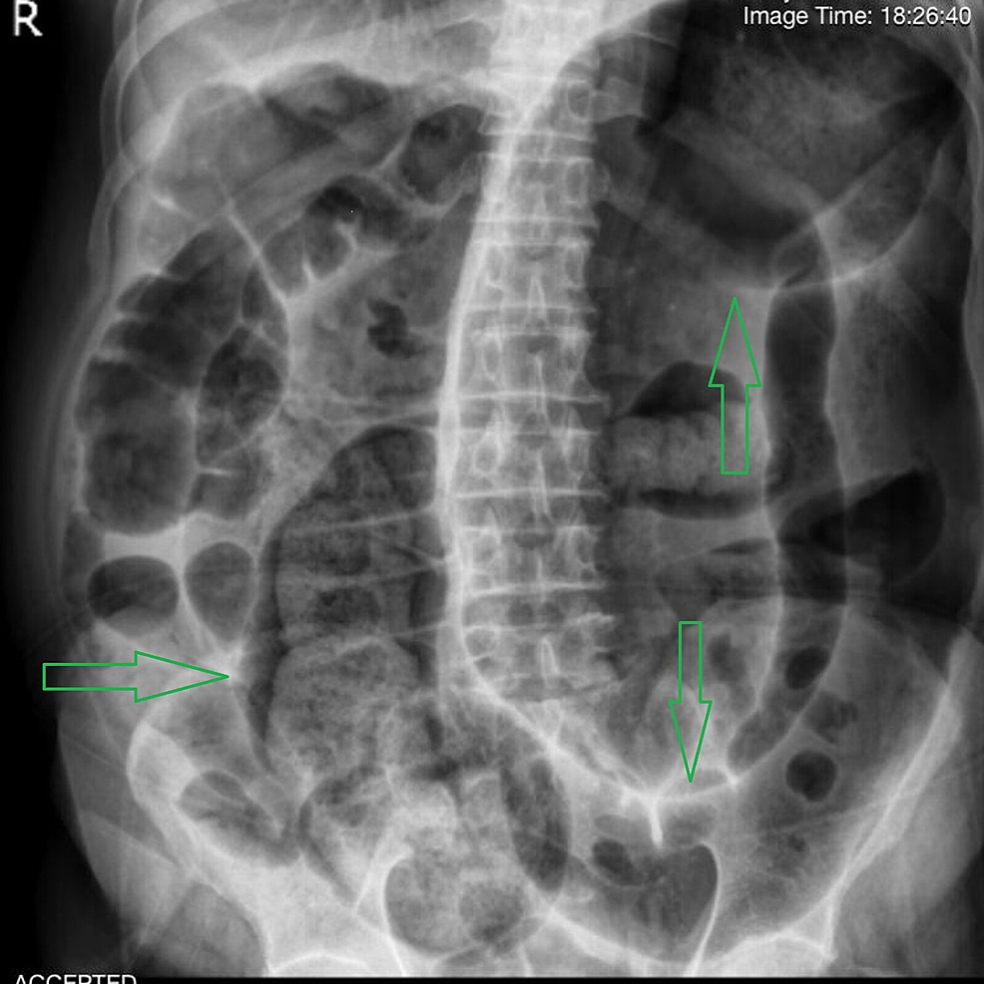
Supine abdominal X-ray Green arrows show dilated bowel loops
